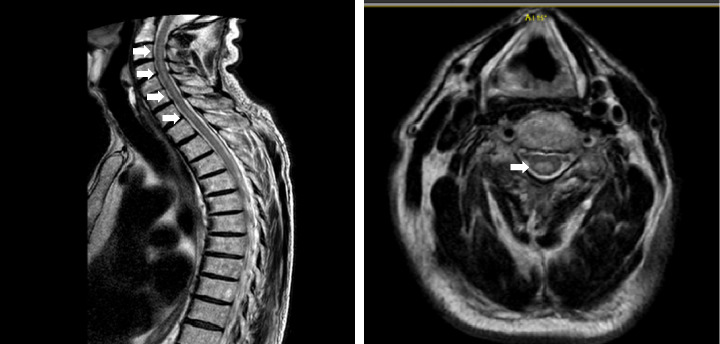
MRI of the spine at presentation (T2 sequence, sagittal and axial), revealing a longitudinal C3-T9 myelitis, shown with white arrows.
